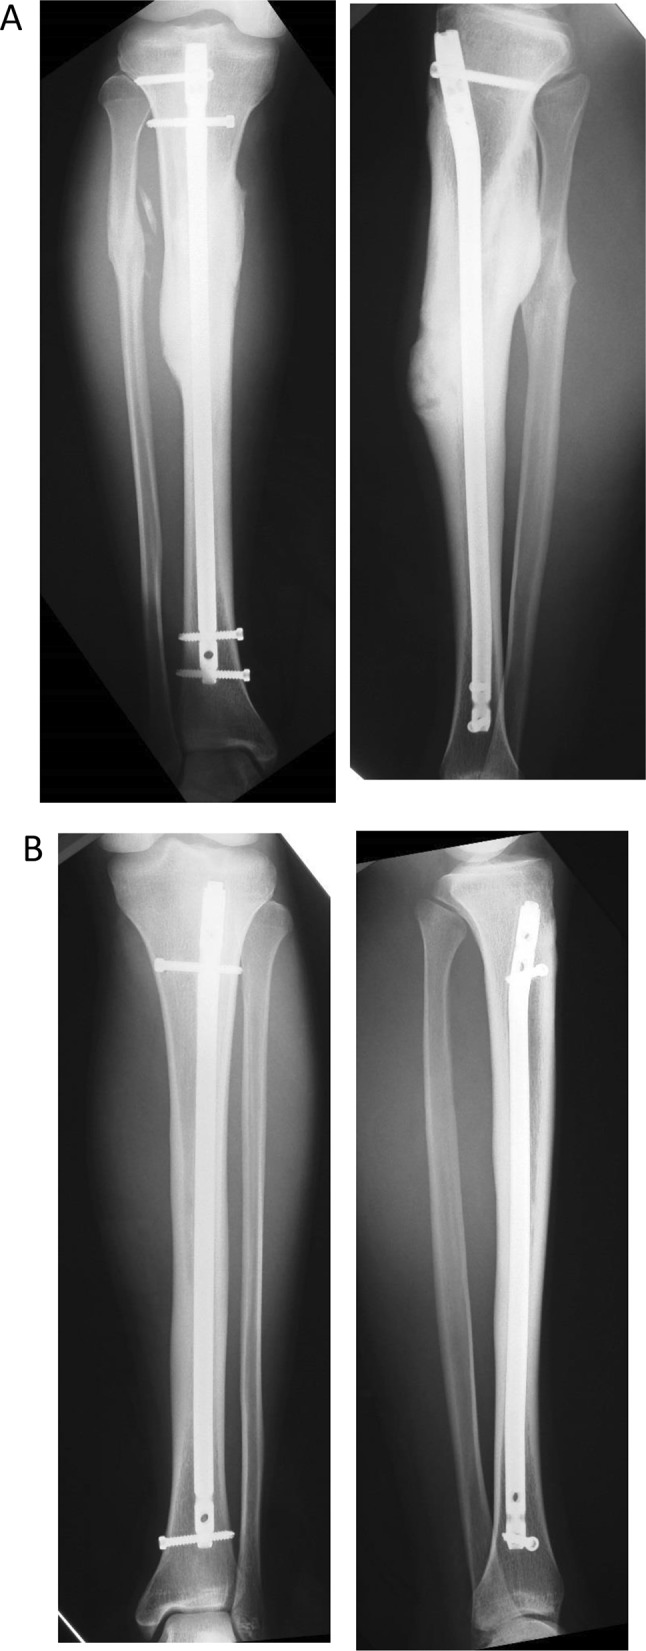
Postoperative antero-posterior and lateral radiographs show newly healed tibial stress fractures. Postoperative radiographs show antero-posterior and lateral low views. The patient was asymptomatic and clinical healing of the fracture is apparent 10 months after the nailing, and a fracture line is hardly visible on the radiographs. A Right side; B left side
